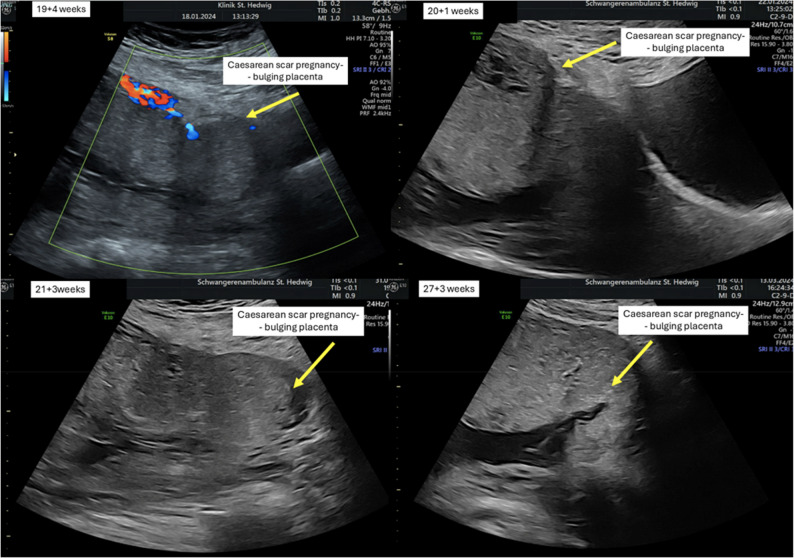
Abdominal ultrasound images demonstrating the bulging placenta at different gestational ages
